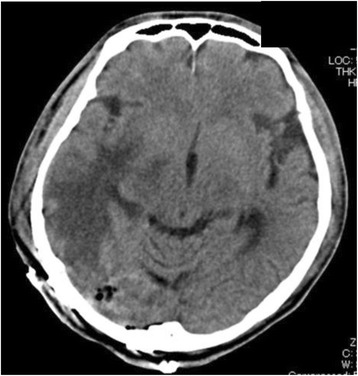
Head CT after removal of the brain tumor. Significant brain edema was confirmed in the head CT after the surgery although the right temporooccipital tumor had been removed
